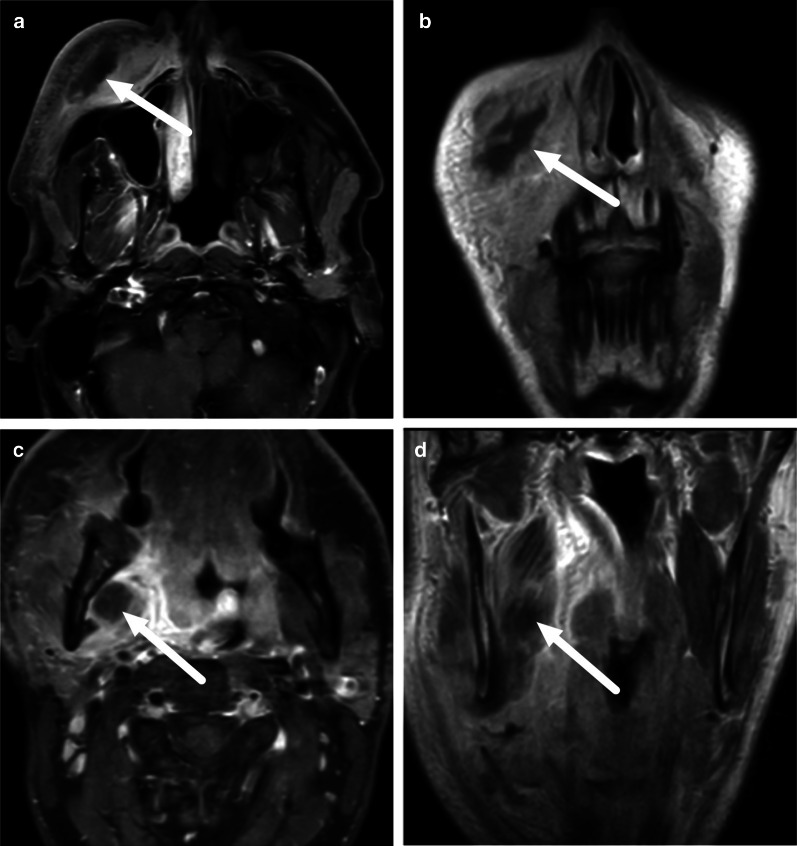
Odontogenic maxillofacial infections in two patients (a and b, c and d). Axial fat-suppressed (a, c) and coronal (b, d) post-contrast T1-weighted images show buccal space (a, b) and masticator space (c, d) abscesses (arrows). Image modified from ref. [11]
